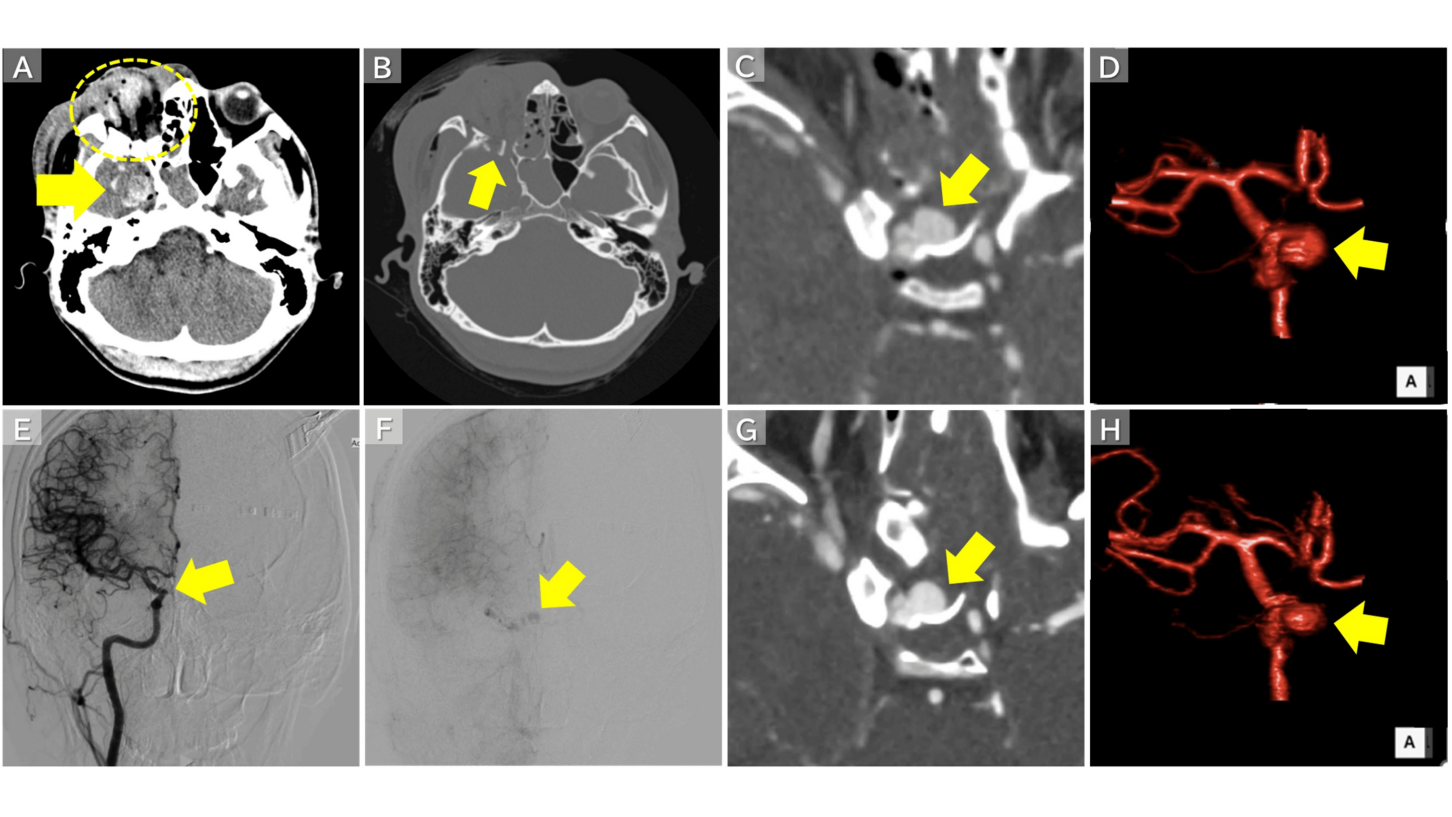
Imaging Findings Prior to Double STA-MCA Bypass and ICA Trapping (A) Right globe rupture (circle) and right temporal lobe contusion (arrow). (B) Skull base fracture (arrow). (C, D) Pseudoaneurysm of the cavernous segment of the right ICA (arrow). (E) The pseudoaneurysm was not visualized on the balloon occlusion test performed the day after injury (arrow). (F) Very faint opacification of the pseudoaneurysm was observed in the late phase. (G, H) CTA on day 3 after injury demonstrated reappearance of the pseudoaneurysm (arrow). STA-MCA: superficial temporal artery-middle cerebral artery; ICA: internal carotid artery; CTA: computed tomography angiography
